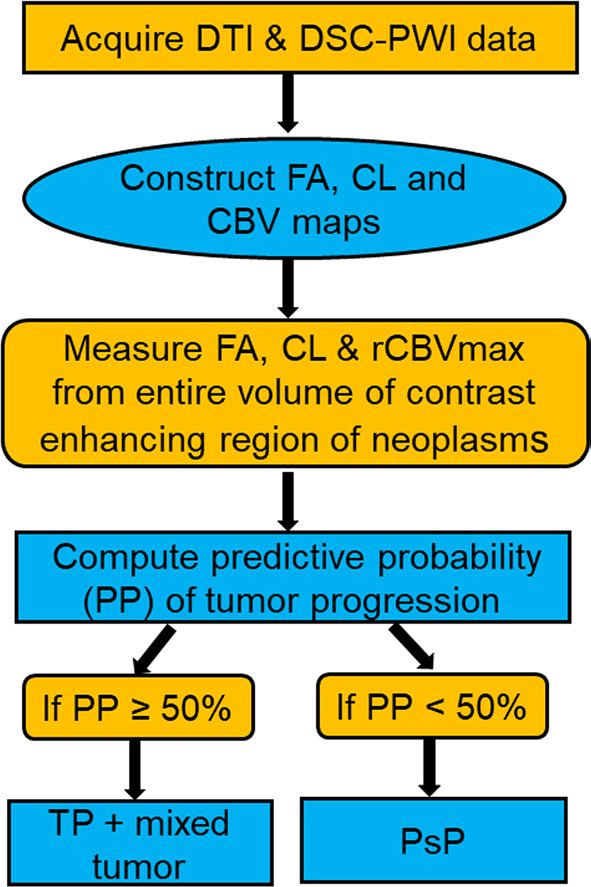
The step-wise process of utilizing multiparametric MRI based prediction model in determining PsP and TP+mixed tumors
